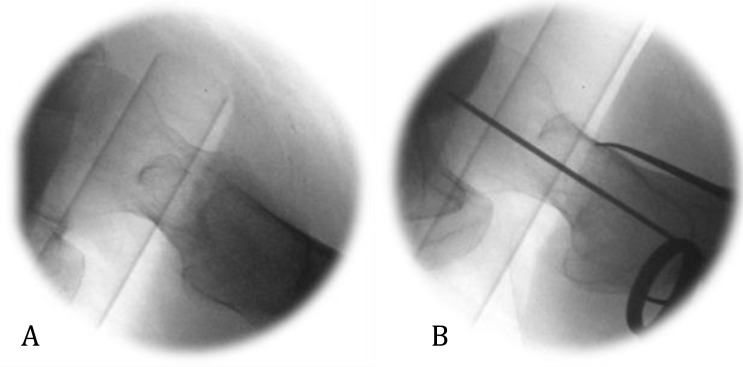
Lateral radiograph of right hip showing (A) apex anterior angulation and (B) correction with downward pressure through a lever placed over the anterior femoral neck.
